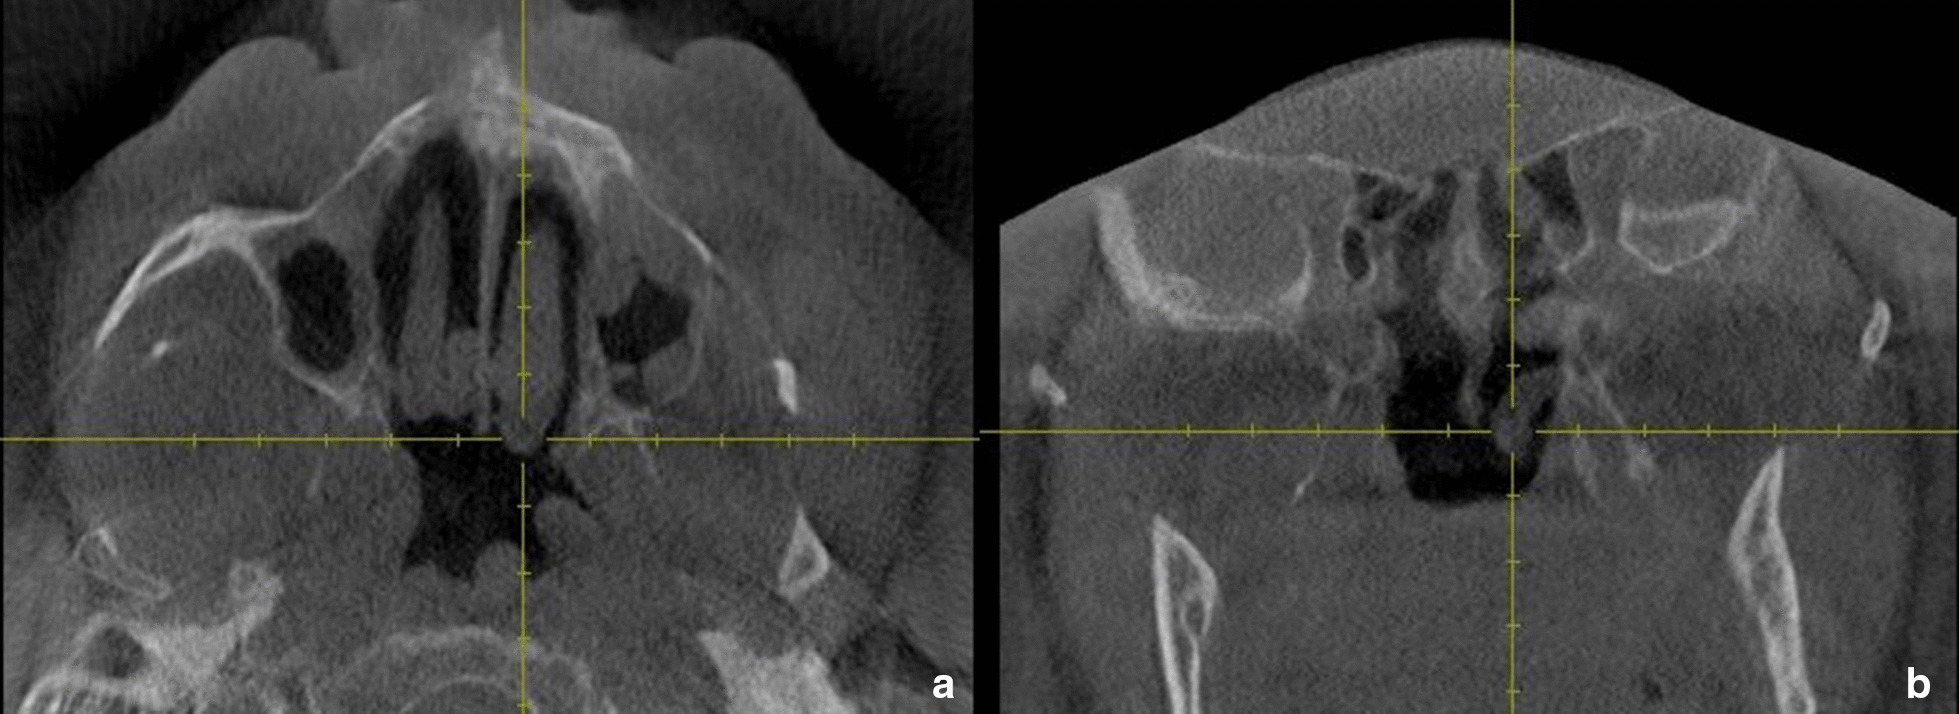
Axial (a) and Coronal (b) CBCT sections showing the site at which the posterior width of the inferior turbinate was determined in the study
